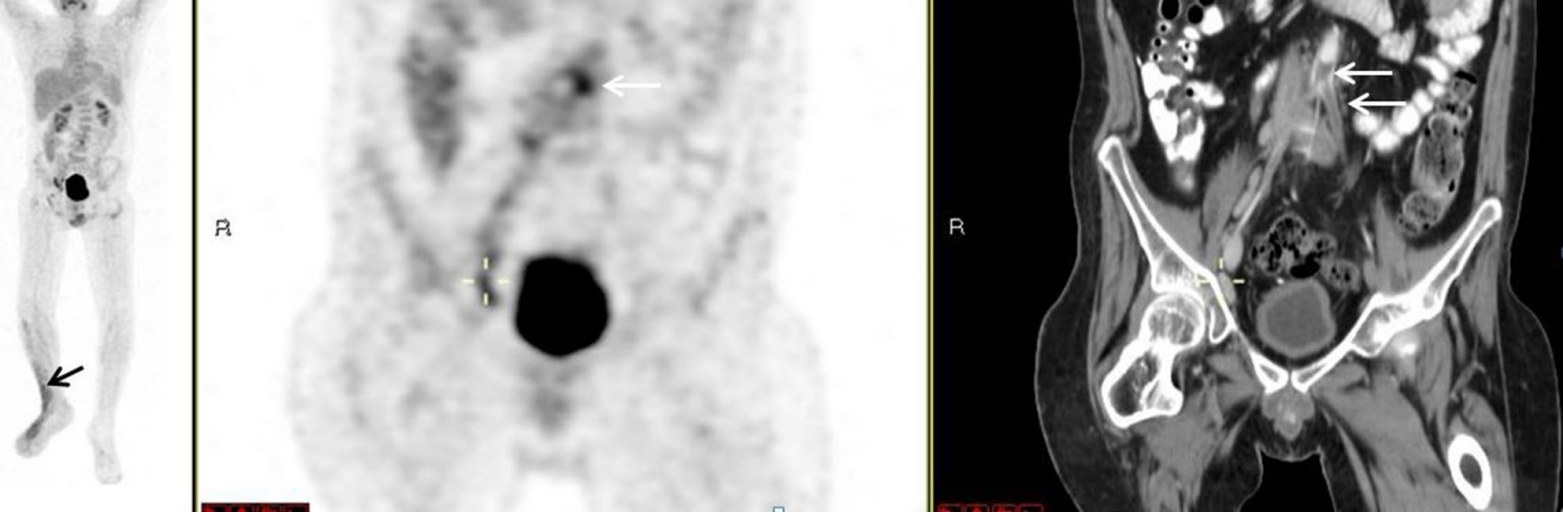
FDG-PET/CT: FDG-PET/CT: maximum intensity projection (MIP) (a) a representative PET (b) and CT (c) coronal slices. A 37-year-old man with Behçet's disease. PET demonstrated increased uptake along the filling defect in the aorta at the bifurcation extending to the iliac arteries (white arrows, b, c) and increased uptake in the soft tissues of the Rt leg (black arrow, a).
